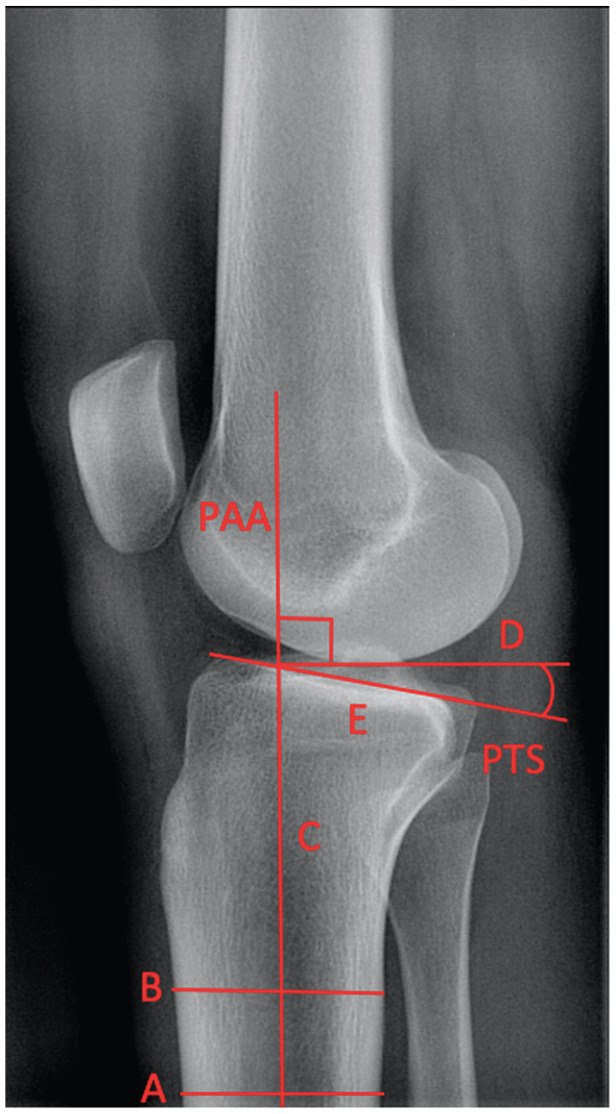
Measurement of the posterior tibial slope (PTS) on a lateral knee radiograph using the proximal anatomic axis (PAA) of the tibia as a reference line. The center point of lines *A* and *B* across the tibial shaft was used to determine the PAA of the tibia (line *C*). A perpendicular line to the PAA of the tibia was drawn (line *D*) as well as a line representing the PTS (line *E*) to allow measurements of the PTS in degrees as the angular difference between lines *D* and *E*.
